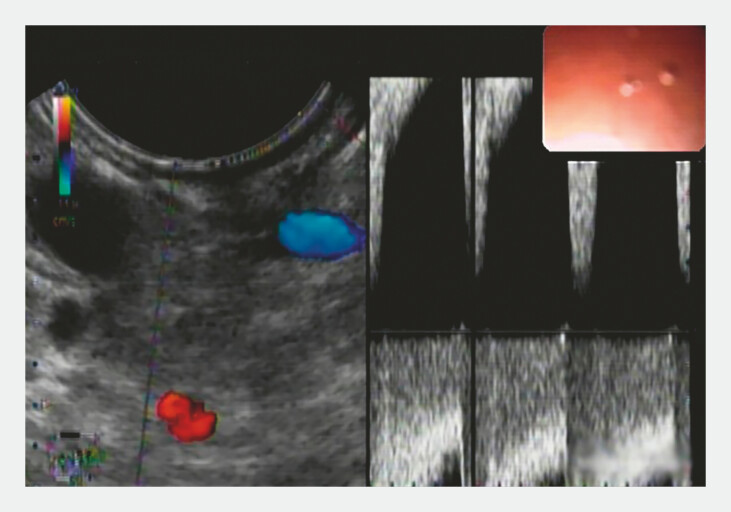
The vessel blood flow was identified to be arterial by endoscopic ultrasound.
